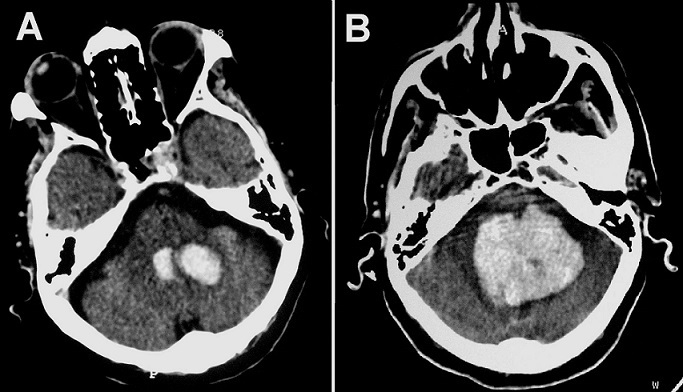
(A): Initial cranial CT-scan showing an acute left hemispheric cerebellar hematoma (about 2 cm in diameter) and a fourth ventricular hemorrhage. (B): Control CT-scan performing about 32 hours of onset revealing an important increase of hematoma volume: diameter about three times as large
